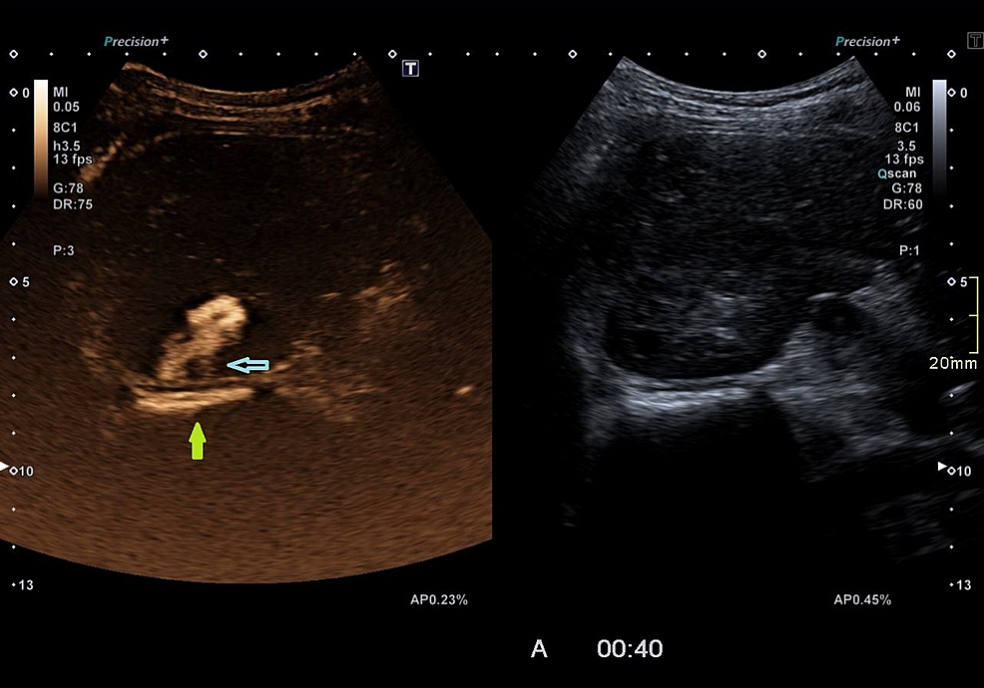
Contrast-enhanced ultrasound (CEUS) retrograde pyelography demonstrating the leak of contrast agent through the renal parenchyma (blue arrow) into the perirenal space (green arrow).
